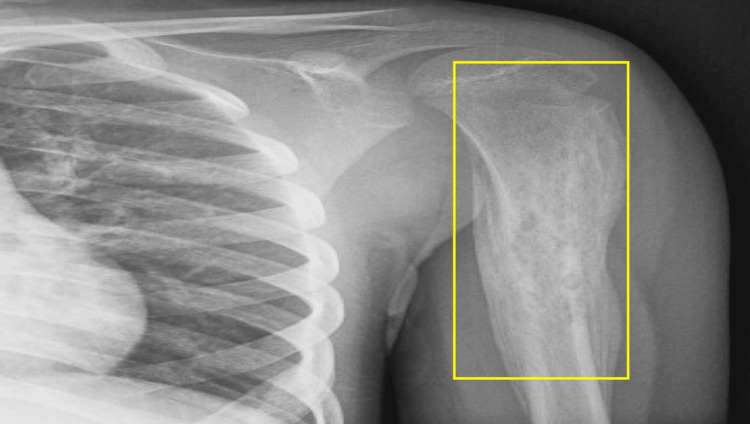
X-ray left humerus showing permeative mixed sclerosis and lucency and an extensive multilayered periosteal reaction
